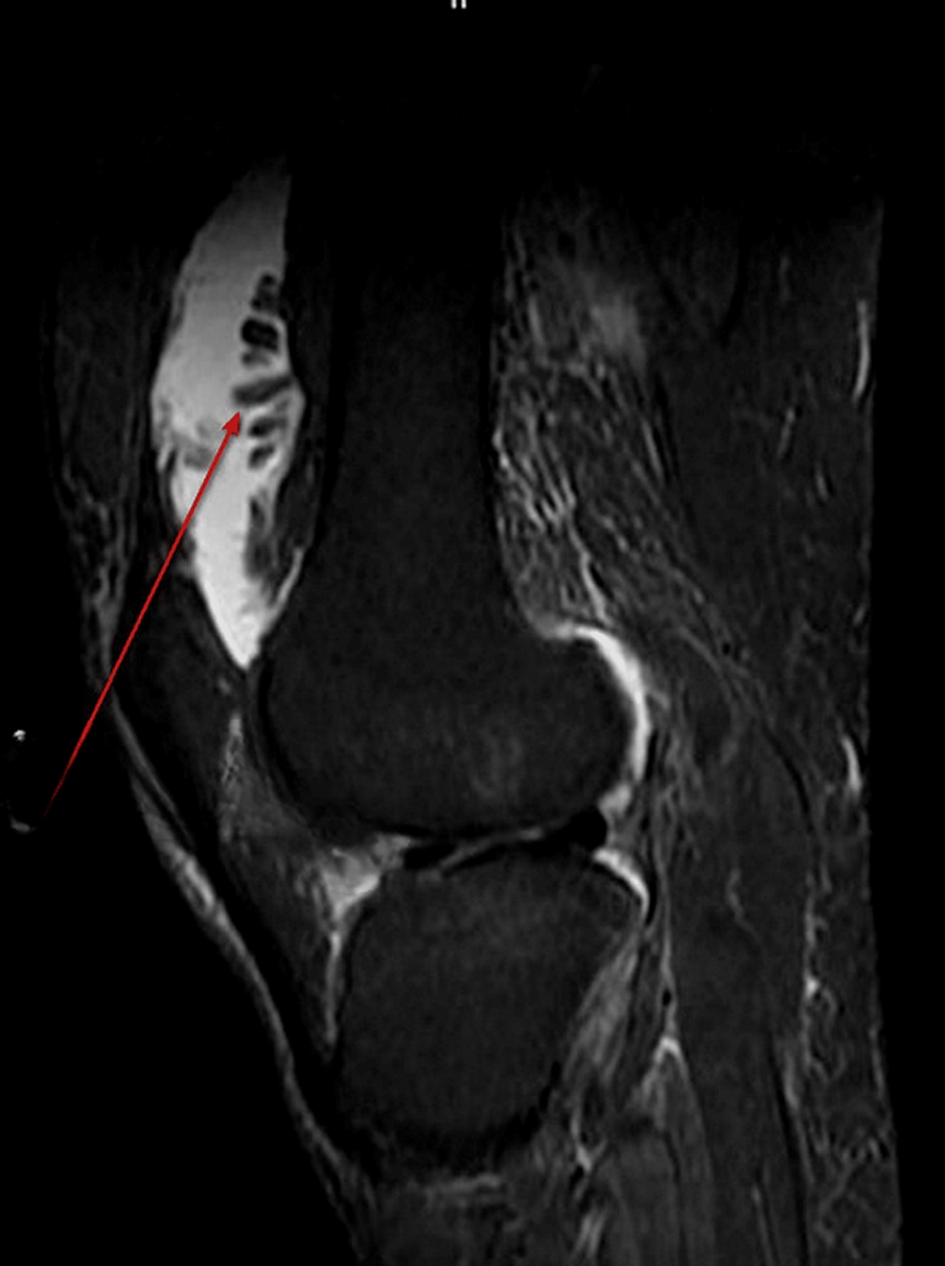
Sagittal STIR MRI showing fat suppression in frond-like projections (red arrow). STIR: short tau inversion recovery, MRI: magnetic resonance imaging
